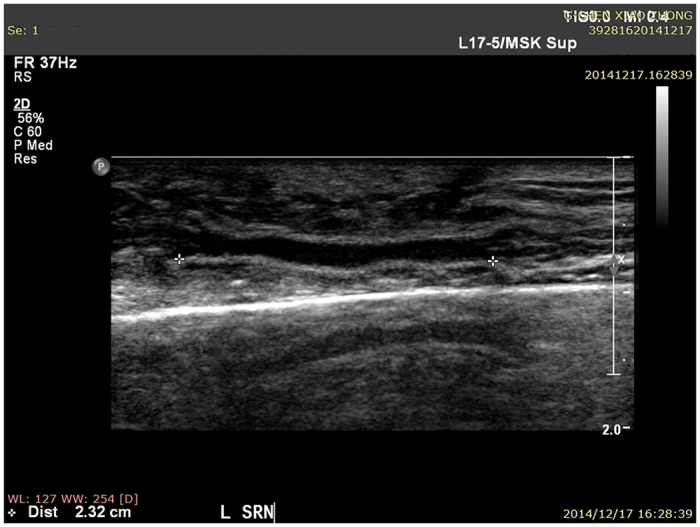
PGLA nerve conduit and regenerated superficial radial nerve in high-frequency ultrasound at one postoperative month.
